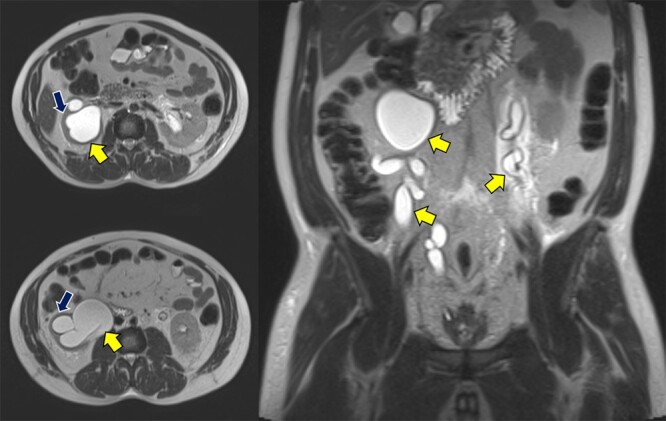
Abdominopelvic MRI T2WI demonstrating severe bilateral hydroureteronephrosis (yellow arrows) with thinning of the right renal cortex (blue arrow).
